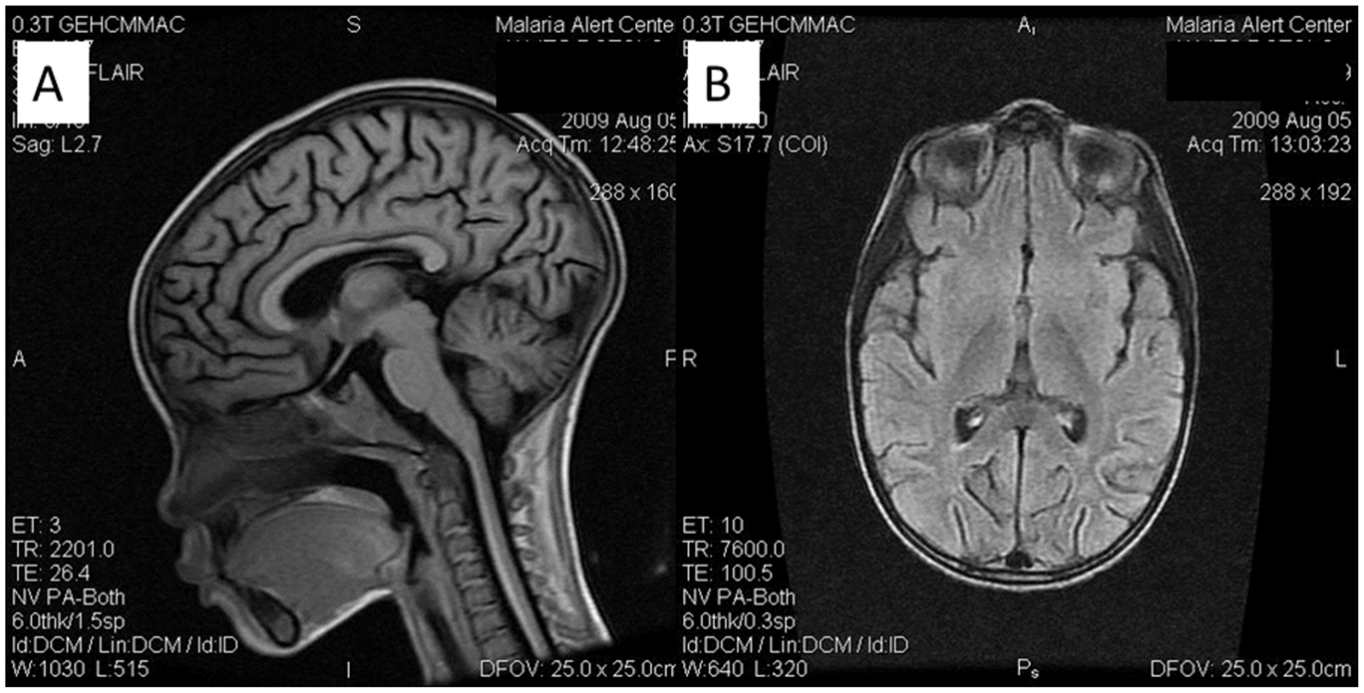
Magnetic resonance imaging (MRI) of a patient with neurologic illness associated with typhoid fever, Malawi. Coronal T1 FLAIR (A) and axial T2 FLAIR (B) MRI Images demonstrating generalized cerebral atrophy, 7 year-old male with neurologic illness associated with acute typhoid fever, Malawi.
